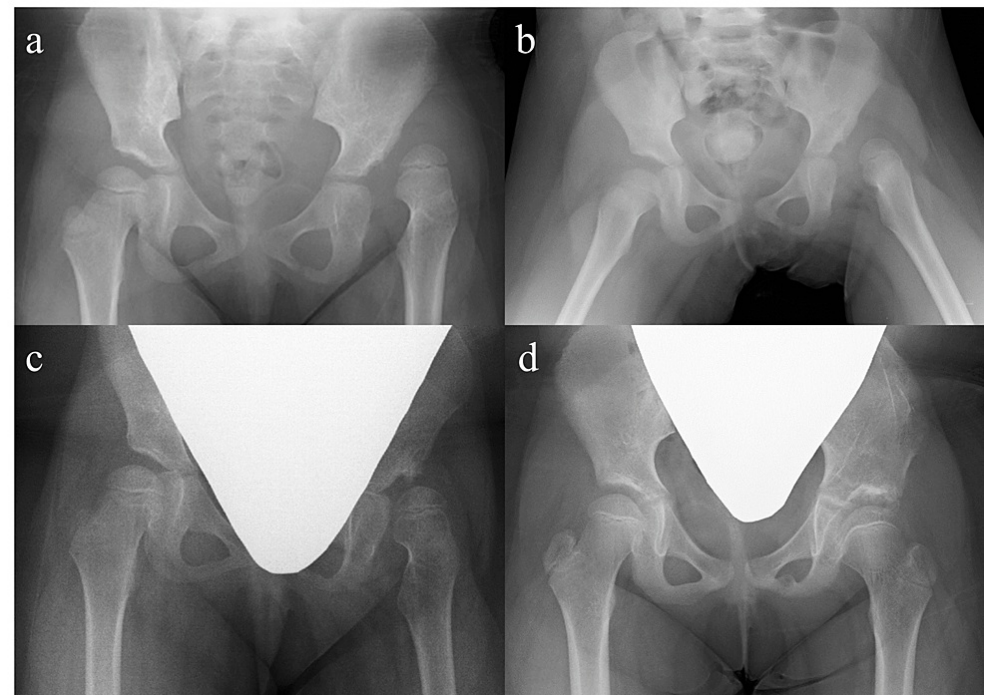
Supine anteroposterior hip radiographs of Case 1. (a) An initial hip radiograph obtained at four years and eight months of age showing a left-sided developmental dysplasia of the hip with Yamamuro’s “distance a” of −5 mm. (b) A follow-up hip radiograph obtained after 37 days of horizontal skin traction showing an acceptable “distance a” of 1 mm. (c) A follow-up hip radiograph obtained after treatment with a flexion-abduction brace showing the residual acetabular dysplasia of the left hip. (d) The latest hip radiograph obtained four years after Salter innominate osteotomy demonstrating a mild coxa magna with a spherical and congruent femoral head of the left hip.
